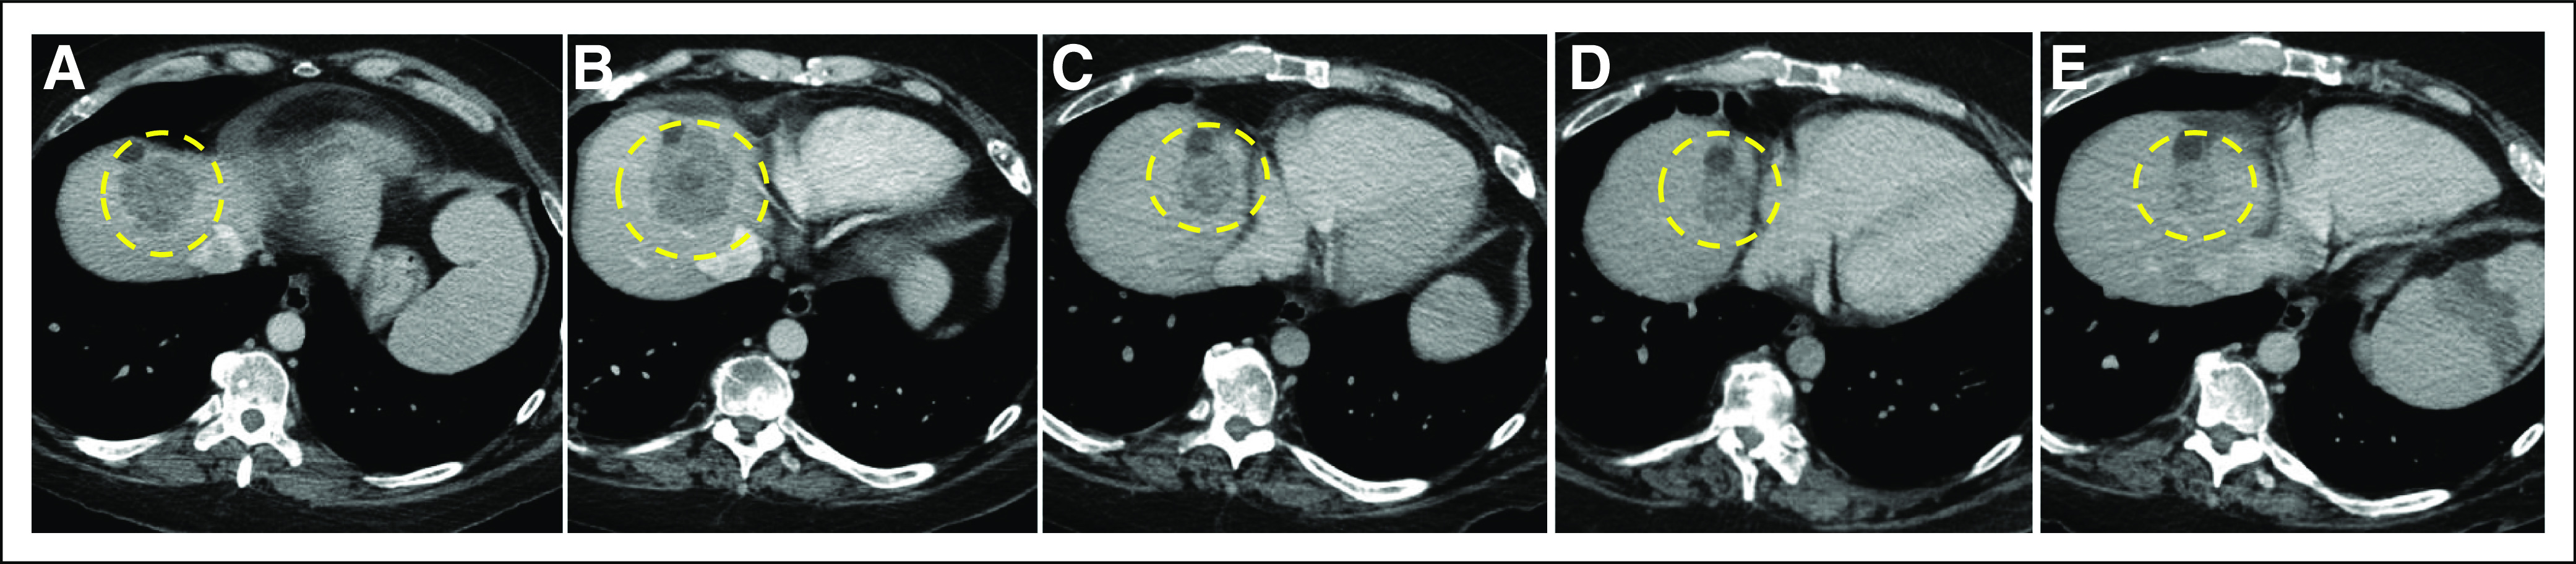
Radiographic evaluation of a hepatic metastasis over the course of treatment. A target metastatic lesion in the patient’s liver was measured by serial computed tomography scans to assess response to treatment. (A) The hepatic target lesion upon conclusion of standard chemotherapy measured 4.8 cm. (B) After 2 months of trastuzumab therapy, the target lesion measured 5.3 cm. (C) After 3 months of treatment with ado-trastuzumab emtansine (T-DM1), the target lesion measured 3.5 cm. (D) After a total of 6 months of treatment with T-DM1, the target lesion measured 3.0 cm. (E) After a total of 12 months of treatment with T-DM1, the target lesion measured 2.2 cm. The dashed gold line indicates the hypodense metastatic target lesion.
